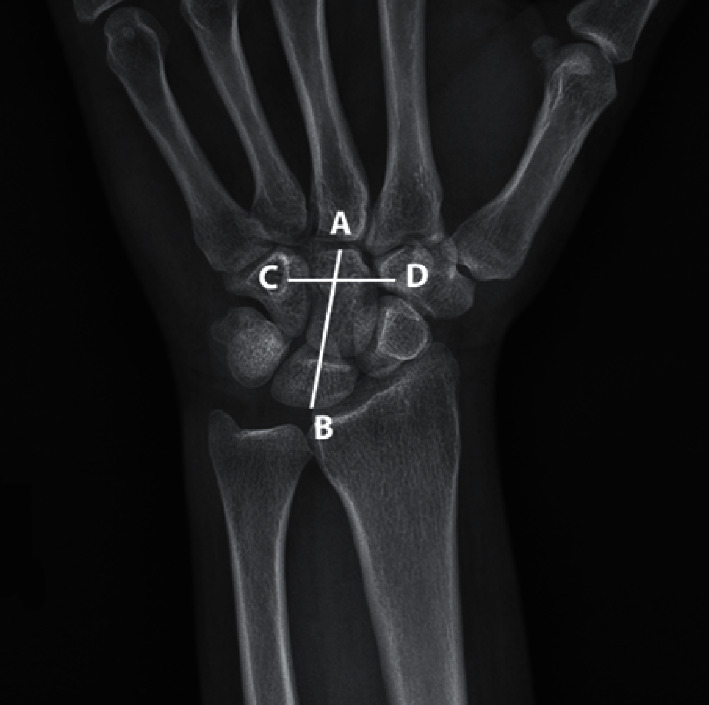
Wrist radiographic criteria. AB, carpal length. CD, carpal width.
